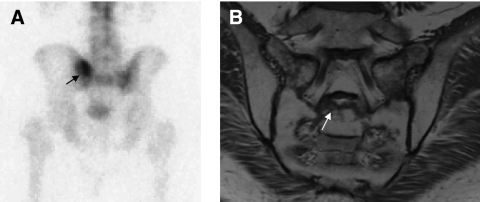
False-positive MDP bone scintigraphy. A male patient with prostate cancer and left sided sacral pain previously treated with IMRT, PSA <0.04. MDP bone scintigraphy (posterior view, A) showed a solitary focal area of uptake in the left side of the sacrum (arrow), interpreted as a bone metastasis. Symptom progression with bilateral sacral pain but PSA remaining <0.04 prompted an MRI, which showed bilateral oedema in the sacral ala (T1W coronal), (B) and a fracture through S2 (arrow) but no evidence of metastasis.
